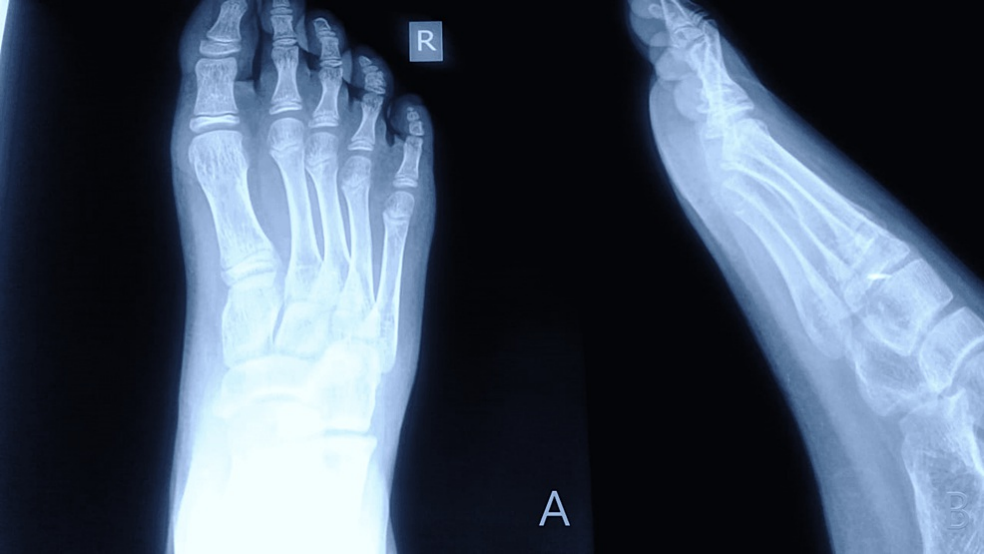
Radiographs at the one-year follow-up Anteroposterior (panel A) and lateral (panel B) radiographs of the foot one year postoperatively demonstrate maintained articular congruity of the fifth proximal interphalangeal joint
